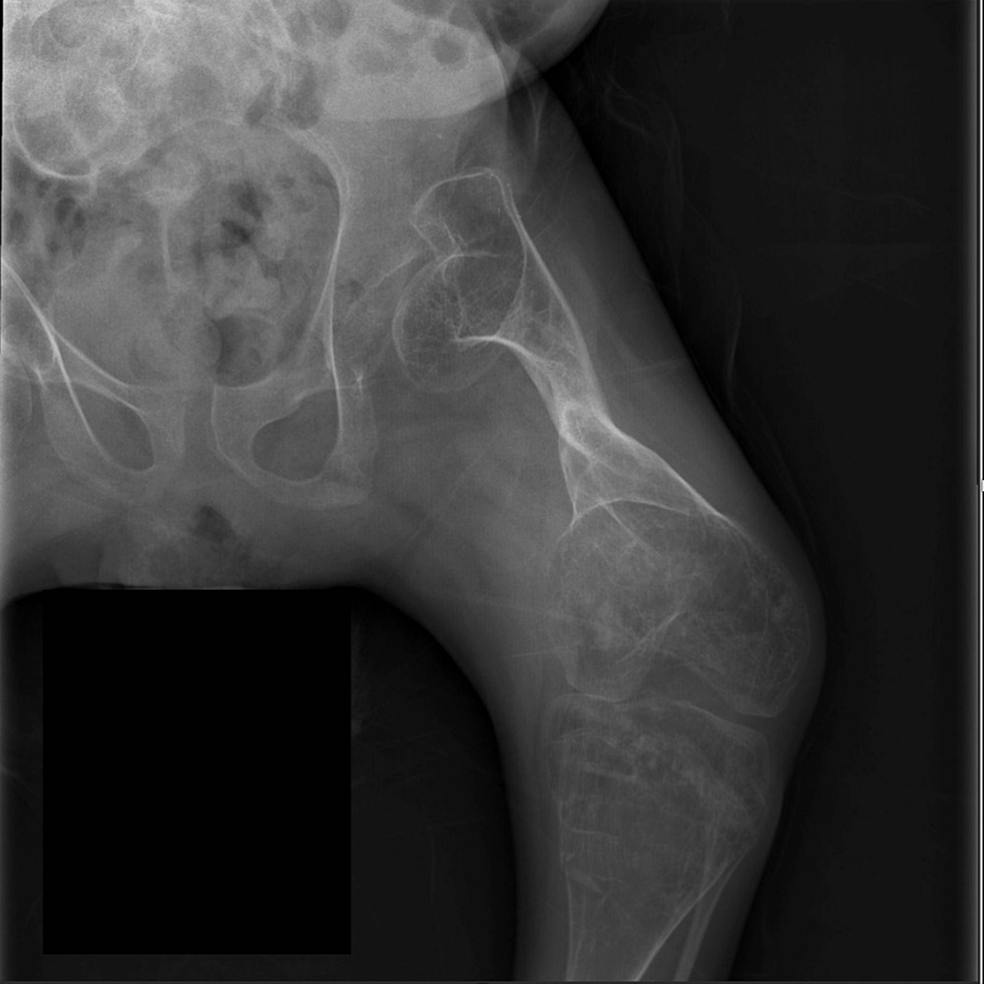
One year postoperative X-ray showed healed left femur osteotomy following rush nail removal
